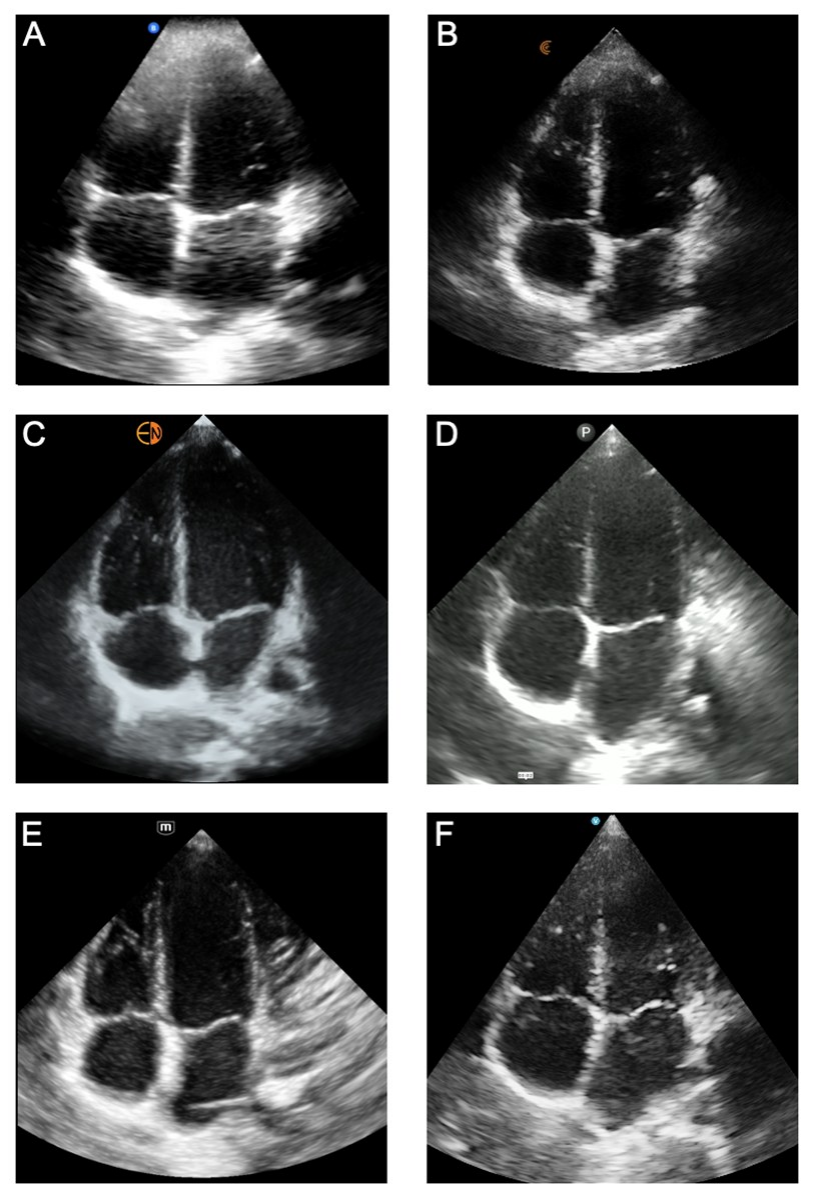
Cardiac Apical 4-Chamber Views acquired from the same pediatric model using six handheld ultrasound devices: (A) Butterfly iQ+™, (B) Clarius®, (C) Kosmos™, (D) Lumify™, (E) Mindray®, and (F) Vscan Air™.
